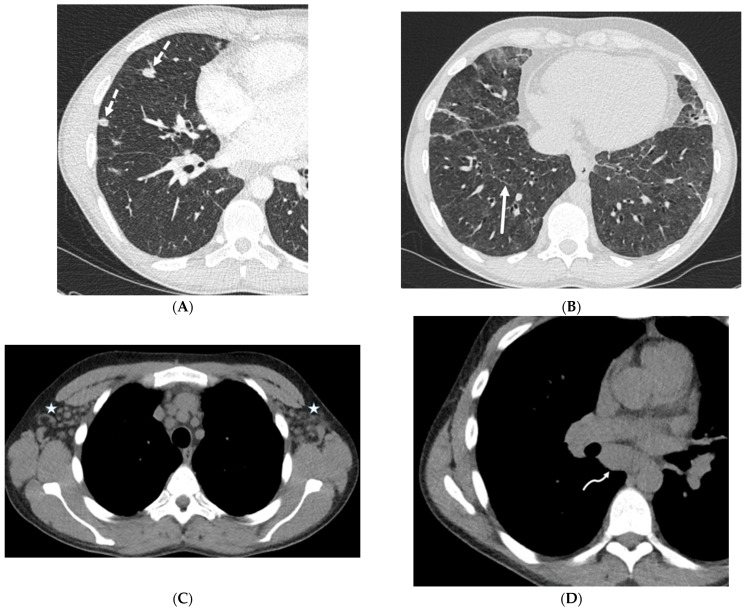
A 35-year-old male with a history of common variable immune deficiency (CVID) complicated by granulomatous–lymphocytic interstitial lung disease (GLILD). Axial chest CT images in lung windows (A,B) show extensive ground glass opacities in lungs with areas of interlobular septal thickening (solid white arrow) and discrete solid nodules (dashed white arrows). Axial chest CT images in soft tissue windows (C,D) show bilateral axillary (stars) and mediastinal (curved white arrow) lymphadenopathy.
